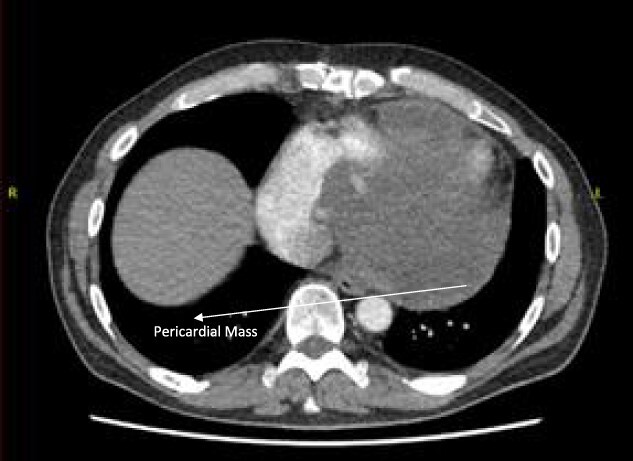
Post-operative CT scan showing pericardial mass following chemotherapy.
